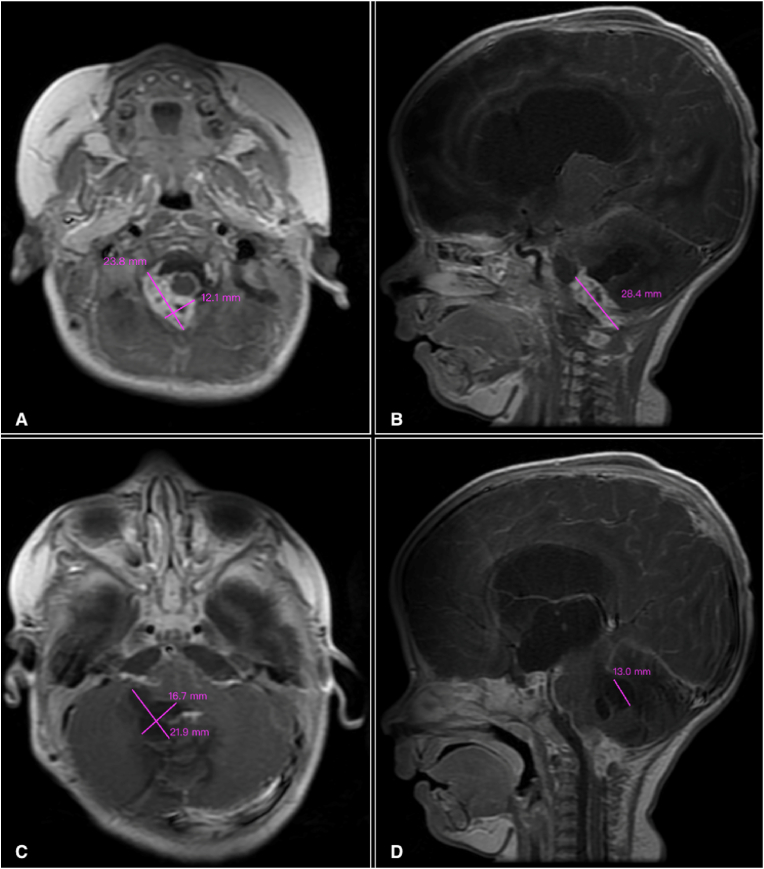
Contrasted brain MRI at 182 days old. (A) Axial view demonstrating heterogeneously enhancing solid mass occupying the right cerebellopontine angle (CPA). (B) Sagittal view demonstrates the mass in the right CPA, the cystic intracerebellar component, and the dilated lateral ventricles. (C) Axial view demonstrating cerebellar multilobulated cystic component. (D) Sagittal view demonstrates the cystic component, the solid component extending through the foramen magnum, and the dilated third and lateral ventricles.
